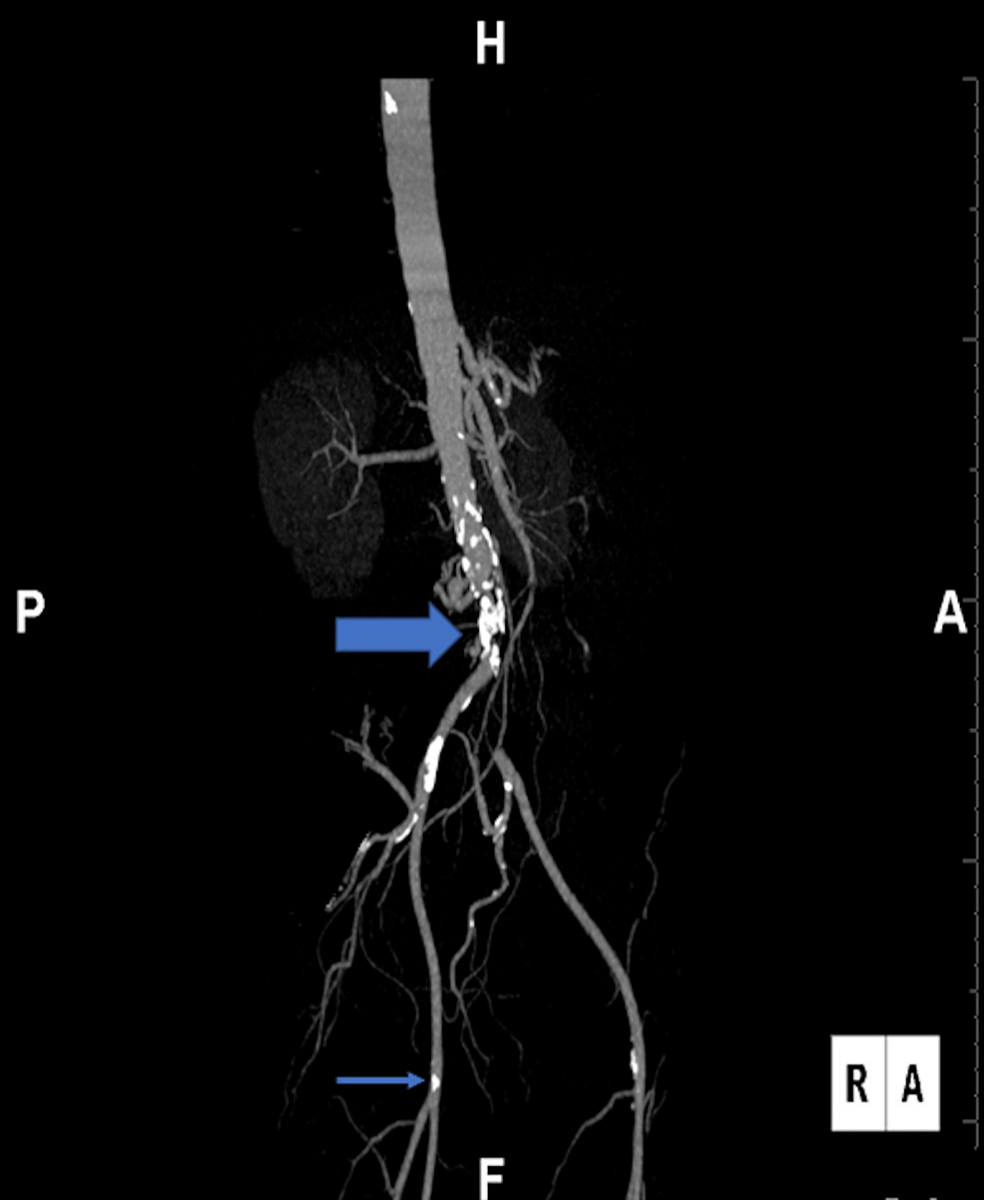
Three-dimensional computed tomography of the abdomen with aortic angiography and lower extremity runoff. Leriche-type occlusion of the distal abdominal aorta and the left common iliac artery (thick blue arrow). A 10 cm occlusion of the right popliteal artery (thin blue arrow) and below-knee trifurcation, with reconstitution of the proximal anterior tibial and mid-posterior tibial arteries.
